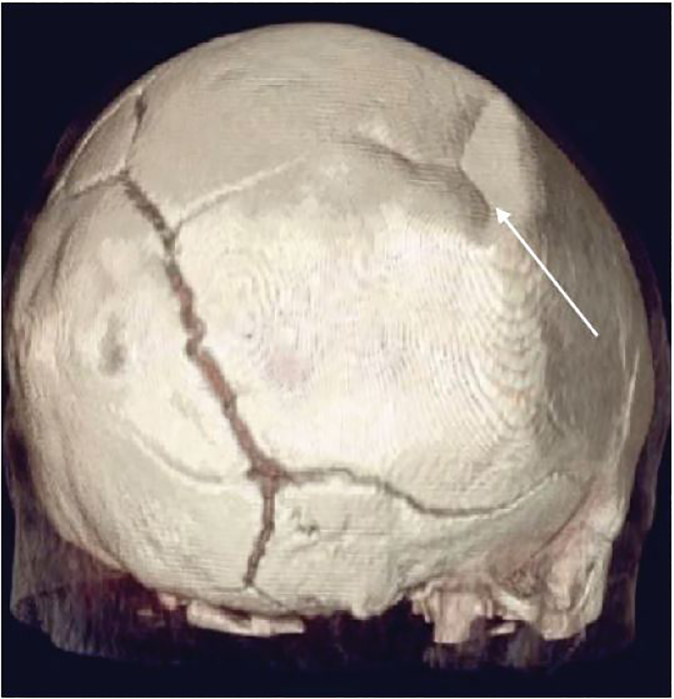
Corresponding computed tomography with three-dimensional reconstruction demonstrating “ping pong” skull fracture.
